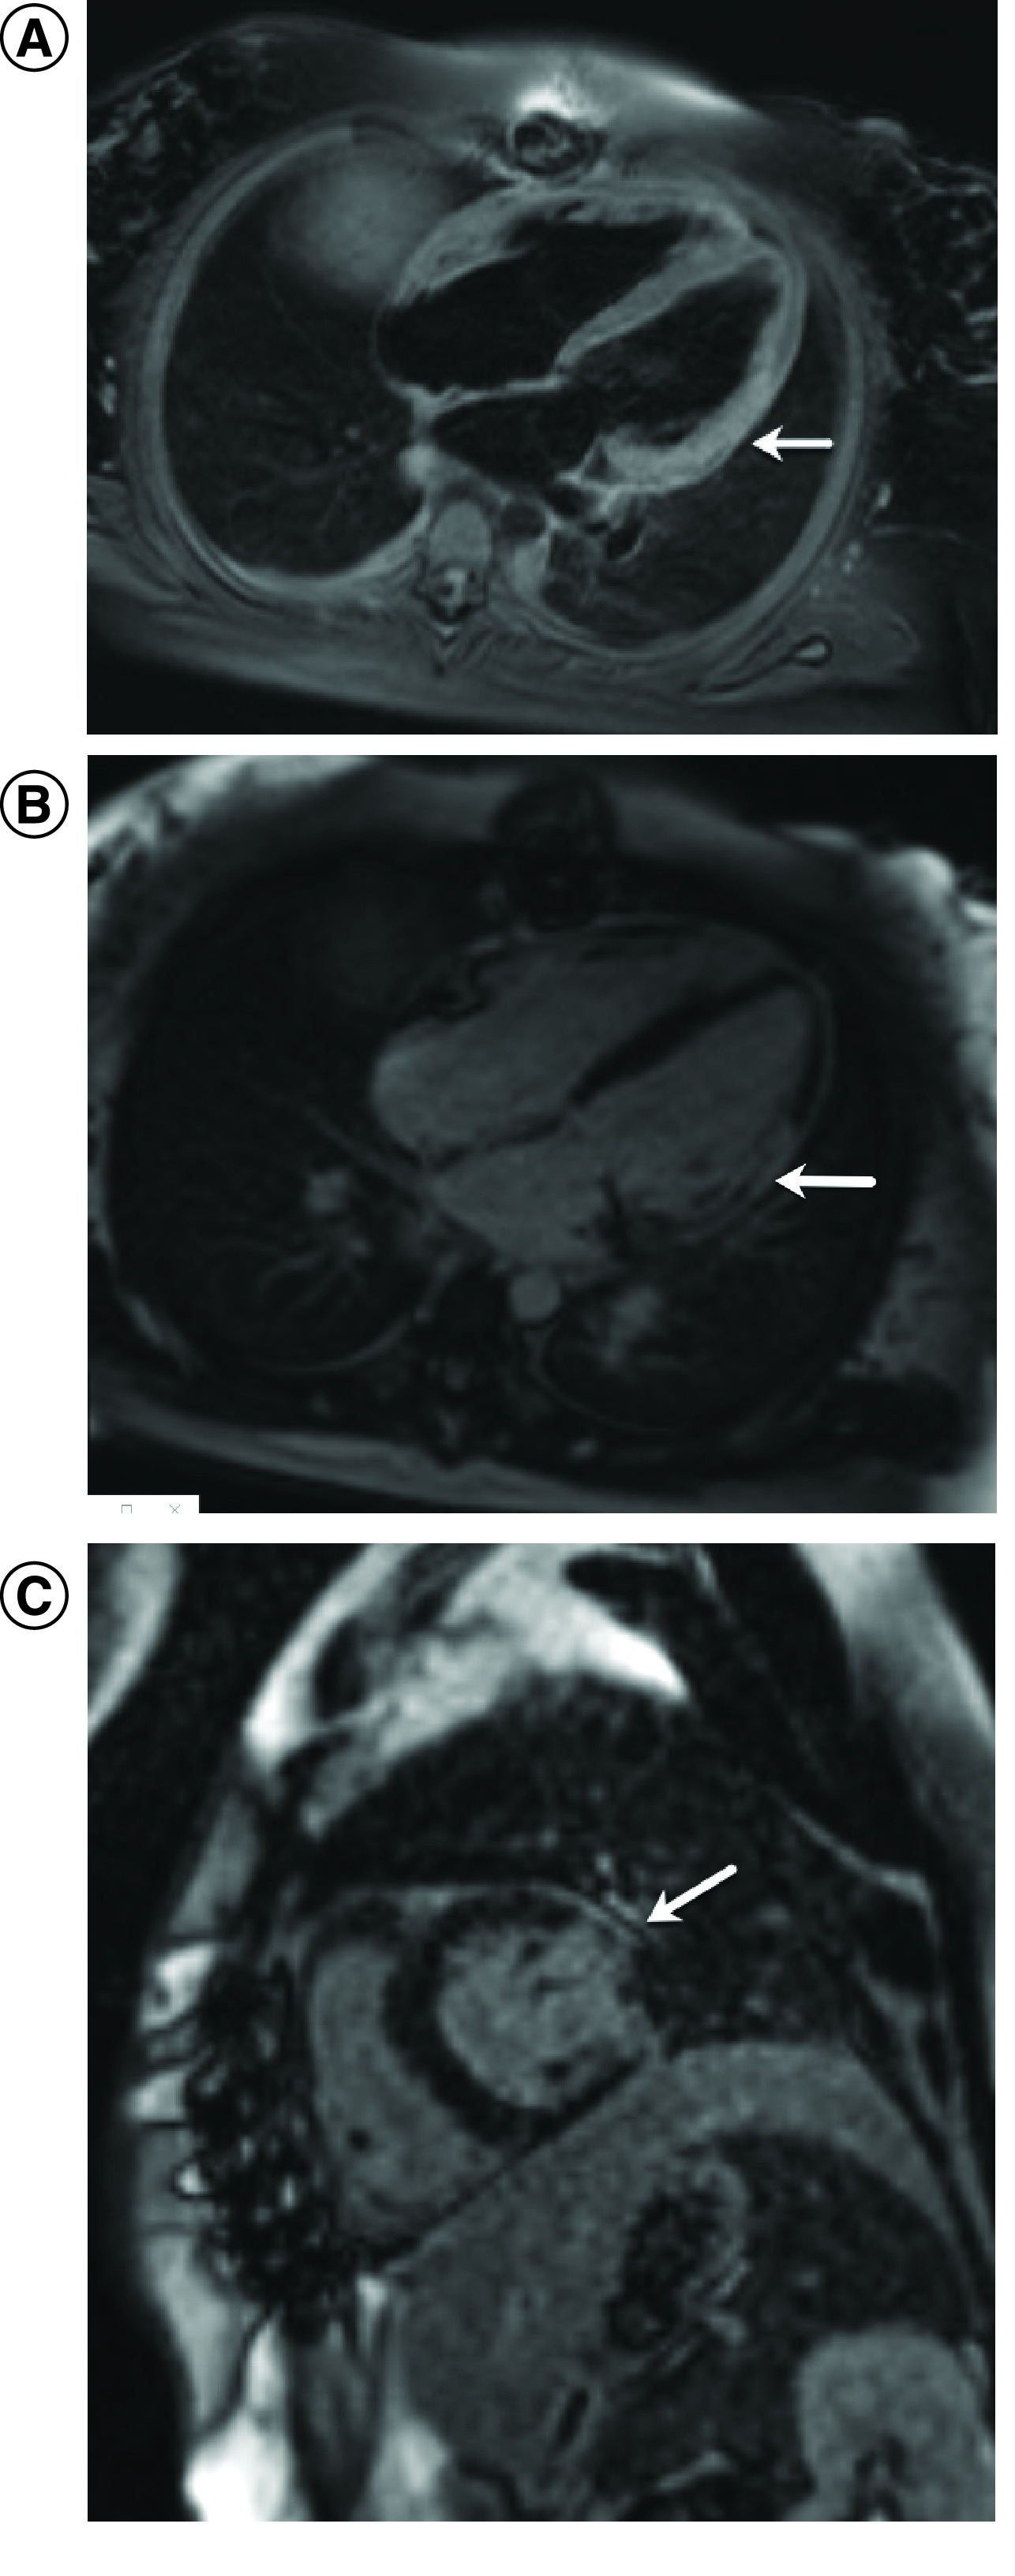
Cardiac MRI findings. (A) CMR showing abnormal increased T2 signal (80 ms) in the lateral wall of the left ventricle indicating myocardial edema. (B) Transmural delayed gadolinium enhancement of basal and mid anterolateral, inferolateral segments. (C) Short-axis view of the CMR showing transmural delayed gadolinium enhancement.
